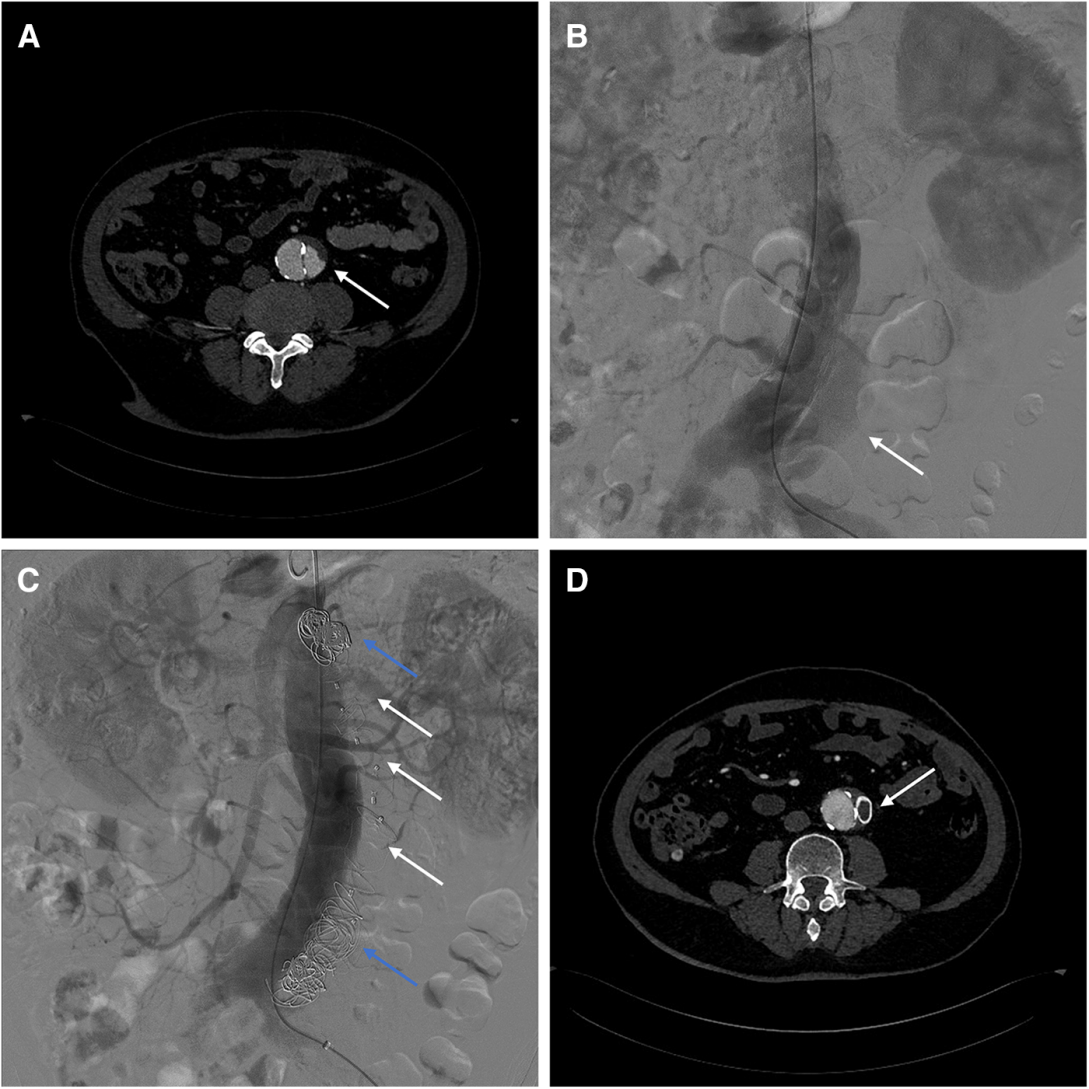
(A) Preoperative CTA showed blood flow in false lumen of aorta. (B) Intraoperative angiography showed the false lumen opacification in abdominal aorta. (C) Angiography showed no opacification of the false lumen after AVPs (white arrow) and coils (blue arrow) placement. (D) 3-month post-surgery CTA shows AVP in abdominal aorta.
